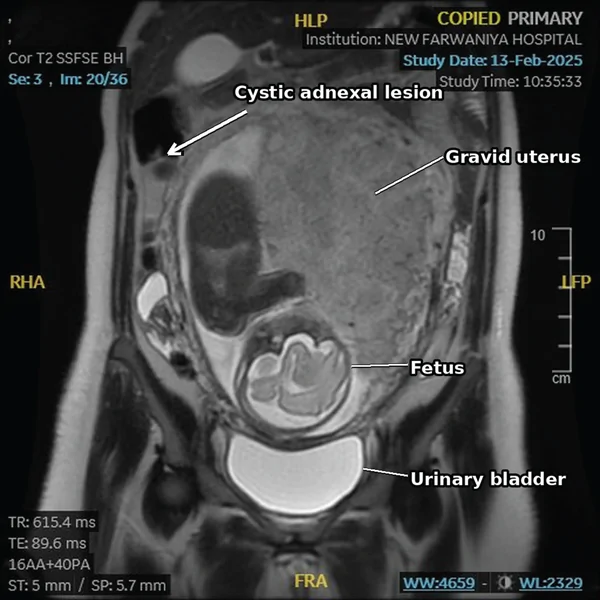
Pelvic MRI, coronal T2‐weighted single‐shot fast spin‐echo sequence. The white arrow indicates the encysted adnexal lesion in the right iliac fossa, reported on the contemporaneous radiology examination to measure 1.9 × 3.8 cm and to be inseparable from the right adnexa. The gravid uterus, the fetus and the urinary bladder are labelled. “RHA” on the left of the image denotes the patient′s right side.
